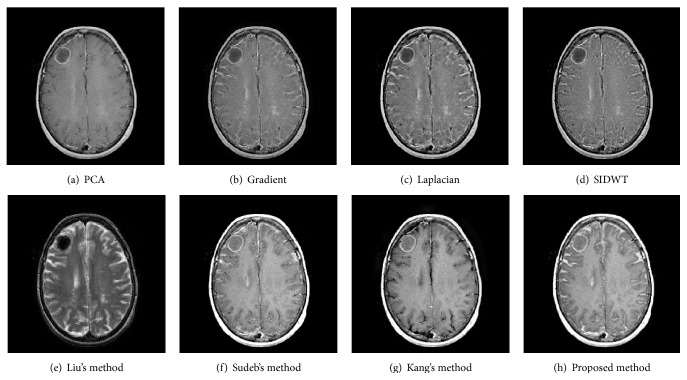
The fusion results of T1-MRI and T2-MRI.
